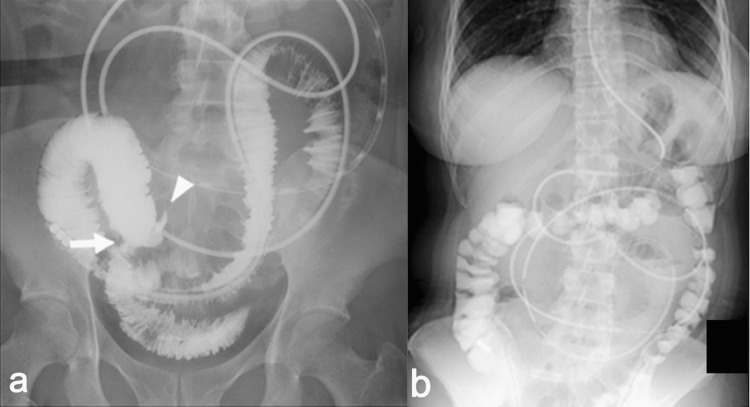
(A) Fluoroscopic X-ray image of WSC injection through the long intestinal tube. Caliber change (arrow) and beak sign-like appearance (arrowhead) were observed. (B) Abdominal X-ray image (standing position) two hours after fluoroscopic X-ray examination showing no WSC within the small intestine. WSC: water-soluble contrast
